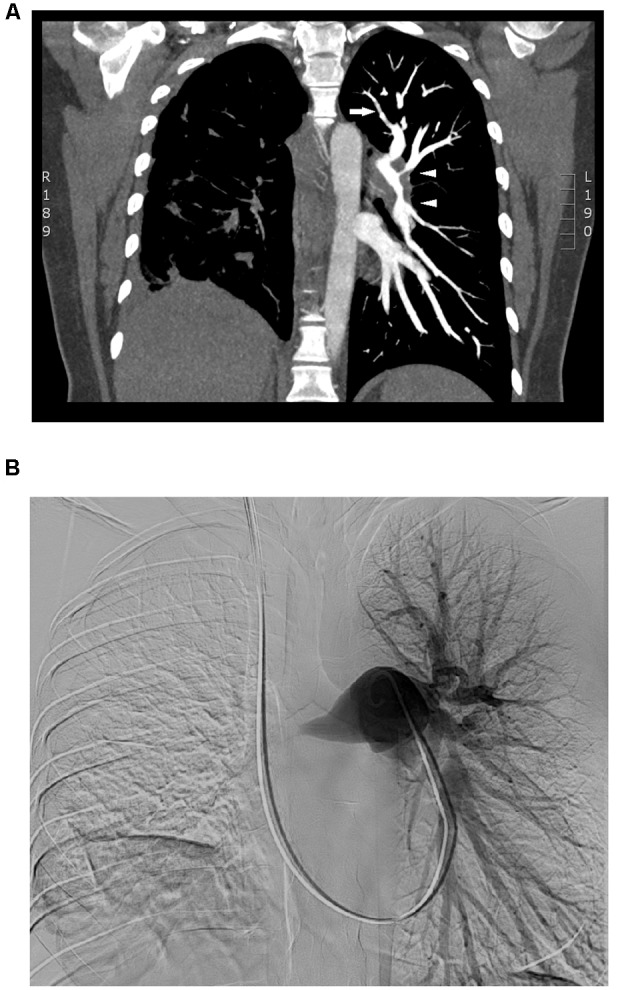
Pulmonary artery occlusion. CT pulmonary angiography (A) showed near-complete occlusion of the right pulmonary artery without any flow and elevated right hemidiaphragm. There was severe thickening of the left lobar pulmonary arteries and short-segment stenosis (white triangles) and wall irregularities (white arrow) in the left lobar and segmental pulmonary arteries. Similar findings were observed during digital subtraction angiography (B).
